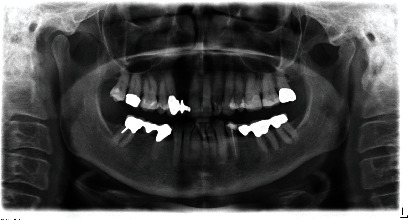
The orthopantomogram (OPT) shows a bone defect in the region of the right lateral incisor.
